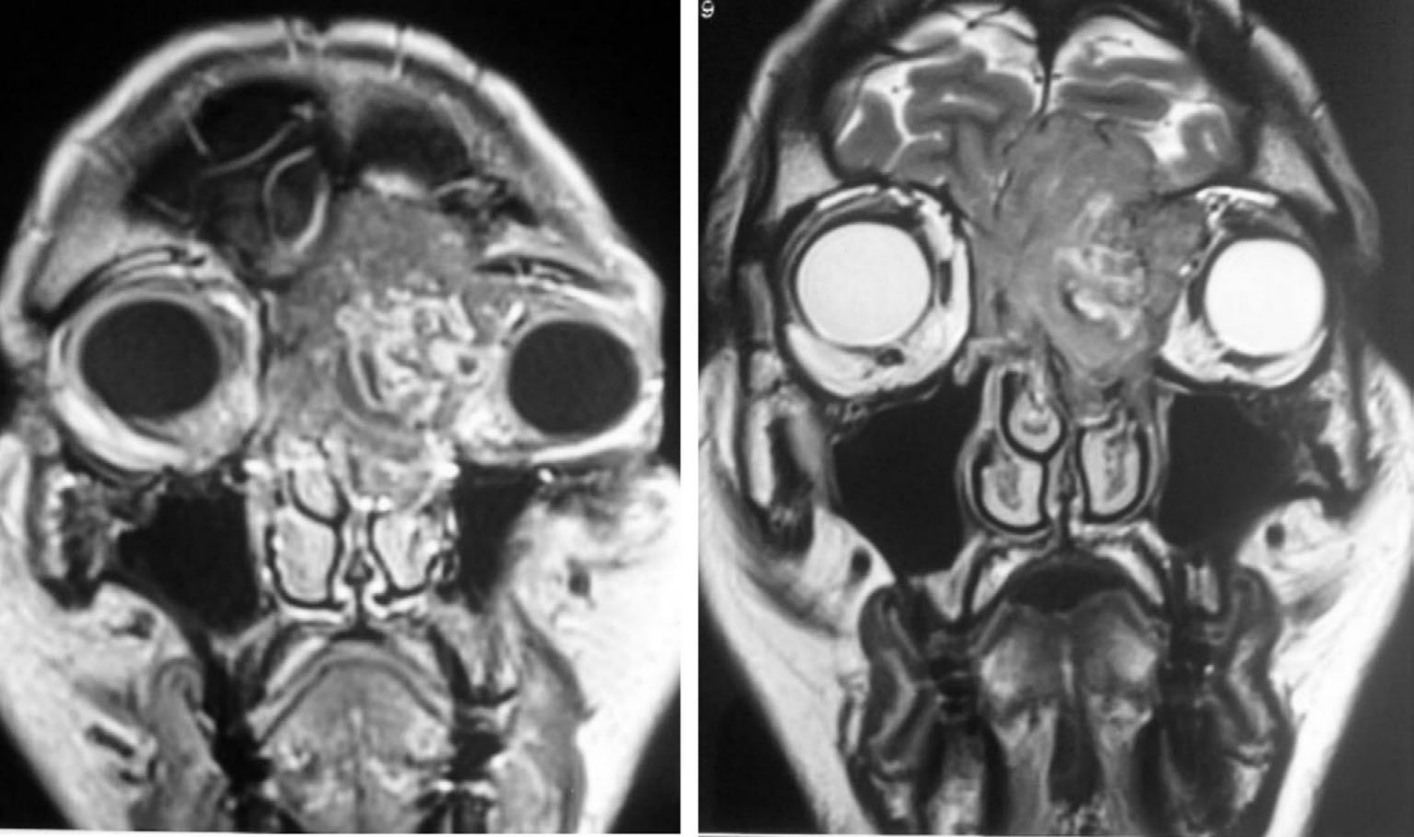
Facial mass MRI in coronal section with T1 and T2 sequences: Tissue process is iso‐intense on T1, showing heterogeneous enhancement with iso‐ and hyper‐intensity on T2, with orbital, intracranial, and intraorbital extension.
